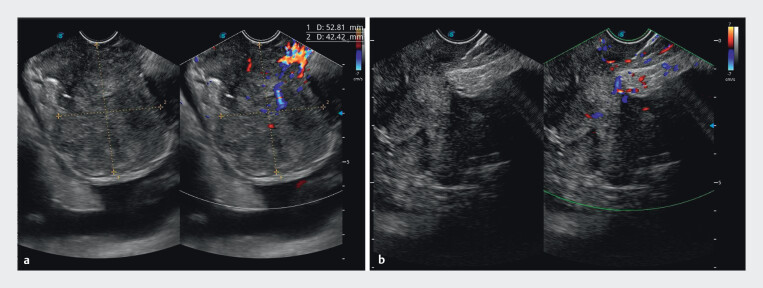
Color doppler flow imaging.
